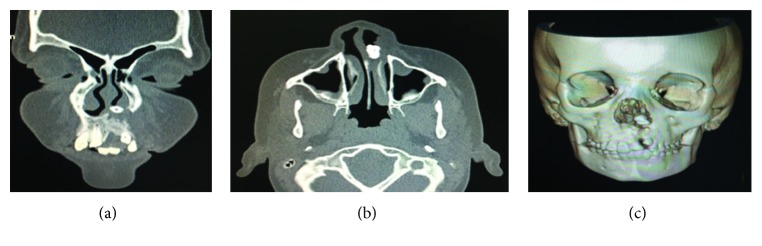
(a, b) CT scan showing intranasal location and soft tissue surrounding the dysmorphic tooth. (c) 3D reconstruction of CT scan showing the ectopic position of element 21.
